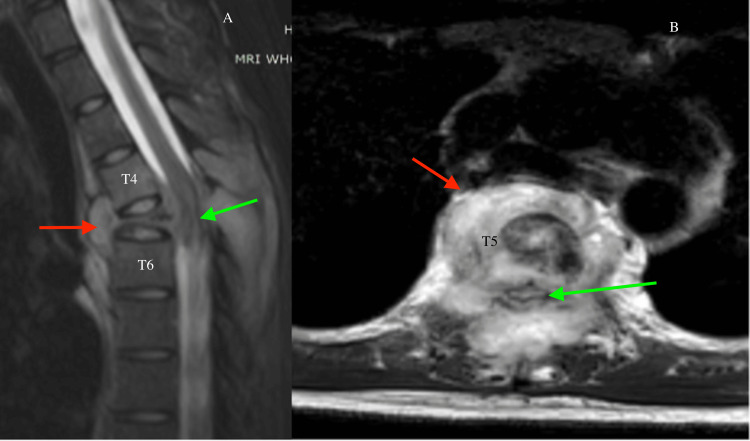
(A) Sagittal T2-weighted MRI and (B) axial T2-weighted MRI show severe destruction of the T5 vertebral body and posterior elements with a surrounding abscess collection. The red arrows point to the abscess collection below the anterior longitudinal ligament and over the T5 vertebra. The green arrows demonstrate the epidural abscess collection at the T5 vertebra with cord edema.
